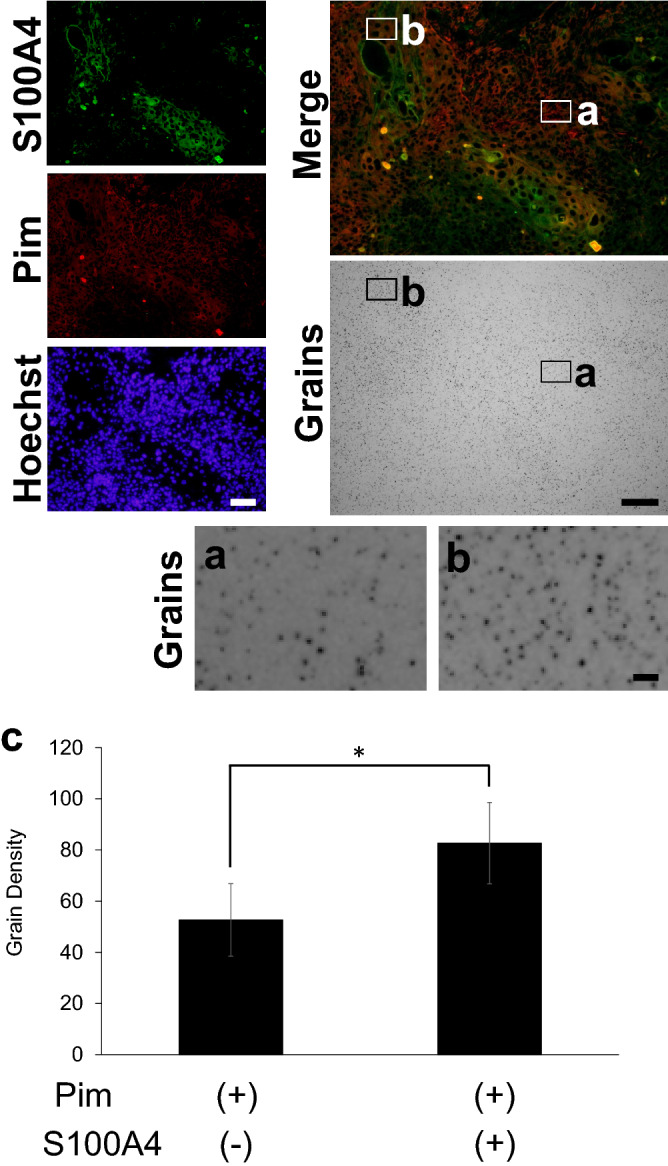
^18^F-FDG accumulation in hypoxic tumor cells expressing human S100A4. (a) Magnified view of micro-autoradiographic image showing the pimonidazole-positive and S100A4-negative area, and (b) the pimonidazole-positive and S100A4-positive area. (c) Determination of grain density in pimonidazole-positive and S100A4- negative or positive area focusing on ROIs of the same size (n = 3, mean ± SEM, Student *t*-test, one-sided, *: *p* < 0.05). Pim: Pimonidazole. Bars: 100 μm (S100A4, Pimonidazole, Hoechst, Merge and Silver grains), 10 μm (a,b).
